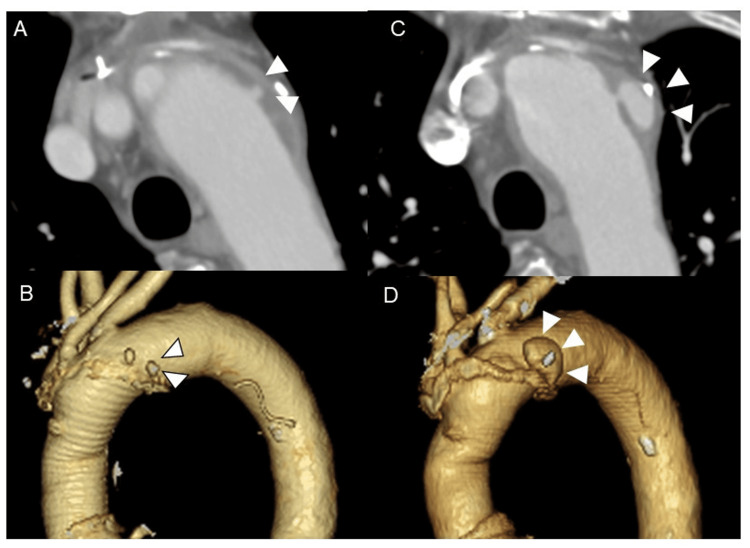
The CT findings immediately after the hemiarch replacement and three months later The CT scans immediately (A, B) and three months postoperatively (C, D) show an enlarged ULP (white arrowheads) from 5 × 5.5 mm to 17 × 9 mm near the distal anastomosis after hemi-aortic arch replacement. ULP: ulcer-like projection; CT: computed tomography
